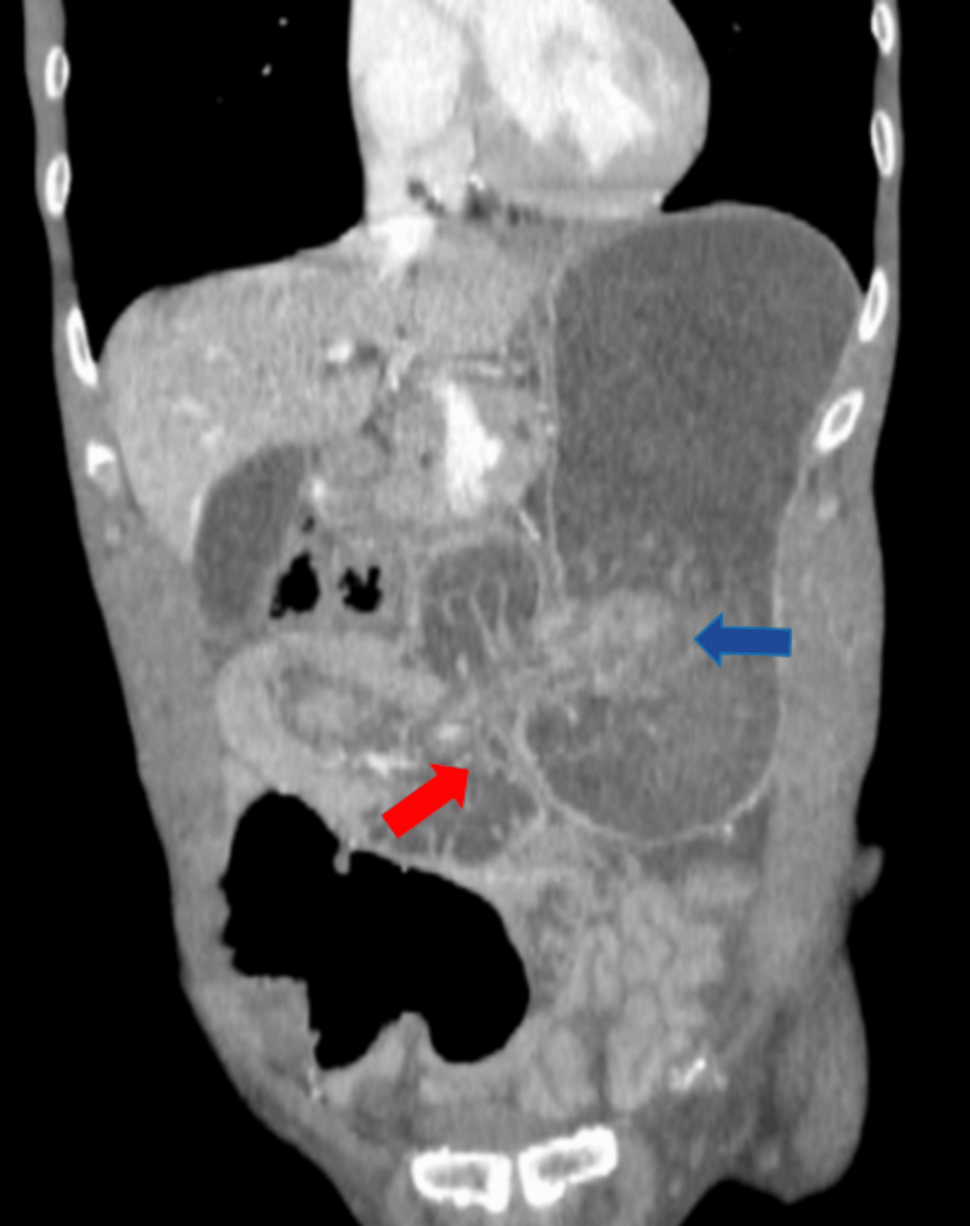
The coronal CECT image shows invaginated jejunal loops within the lumen of the stomach (blue arrow) via a defect in the anteroinferior wall of the stomach in the anteropyloric region (red arrow). CECT: Contrast-enhanced computed tomography.
